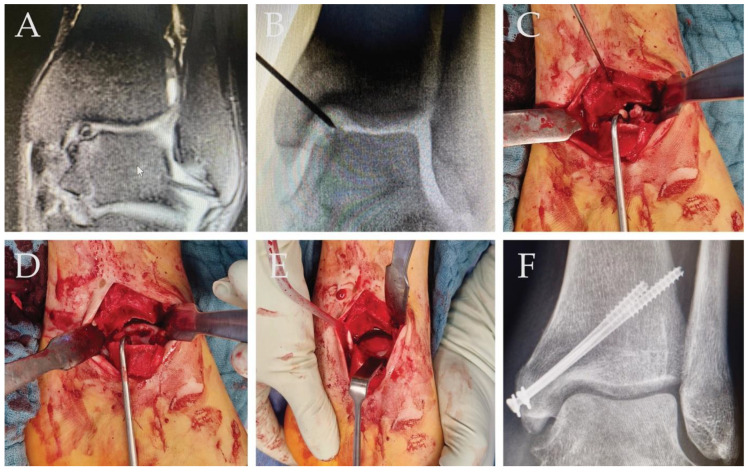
(A) Preoperative MRI Image 4° osteochondritis dissecans of the left medial talus shoulder. (B) Intraoperative X-Ray with the big visible defect. (C) Intraoperative situs, visible free joint bodies. (D) Big defect after clearance of the OD. (E) Defect is filled with iliac crest cancellous bone and covered with collagen hADM membrane; the membrane is fixed with sprayed Fibrin. (F) Six weeks postoperative, good remodeling, and defect in X-ray is not visible anymore.
